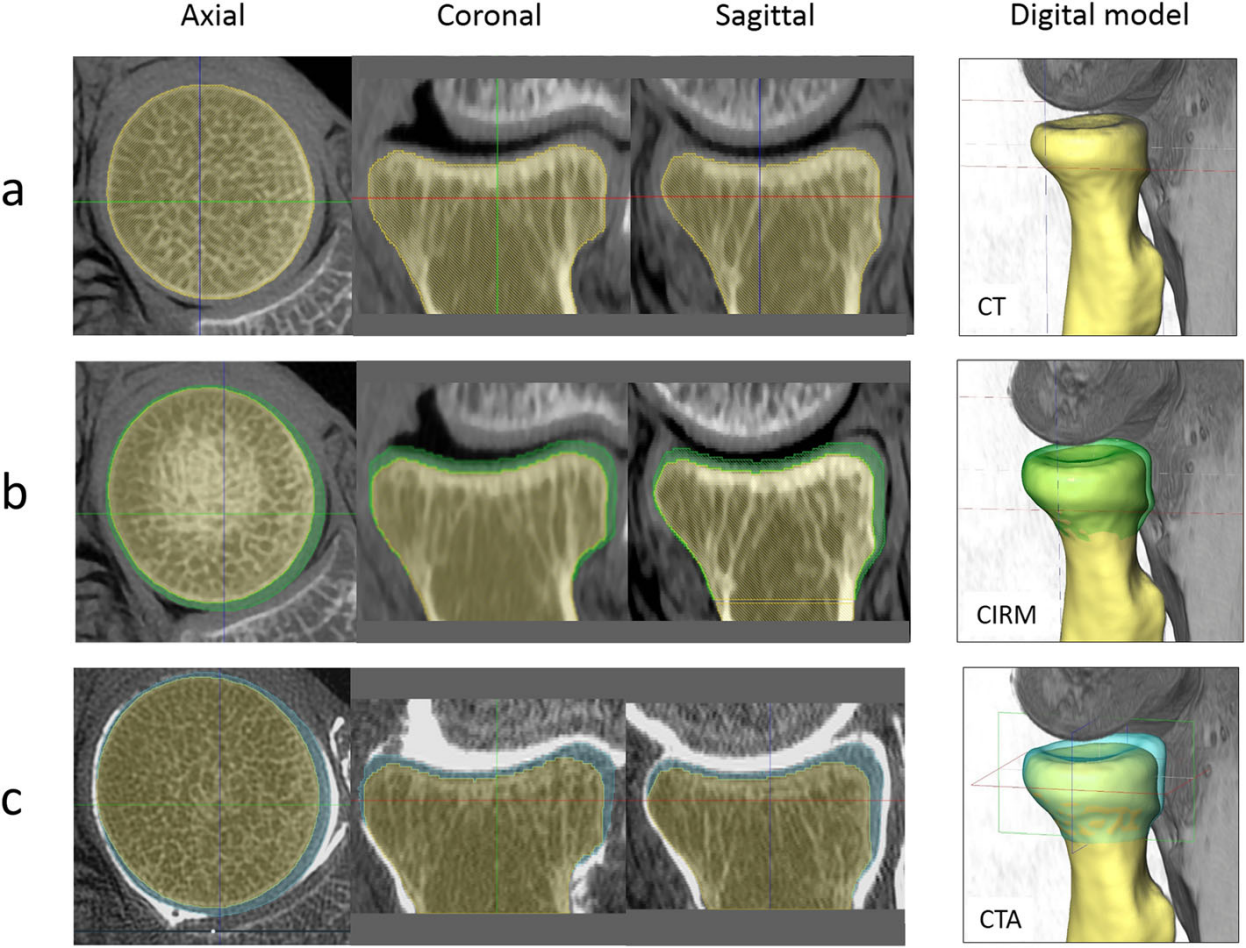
The computerized 3D radial head models of each elbow were generated. a 3D model created from segmentation of the CT images (yellow radial head). b 3D model created from CT images with cartilage-reproducing image reconstruction method (green radial head). c 3D model created  from segmentation of the CT arthrography images (light blue radial head)
